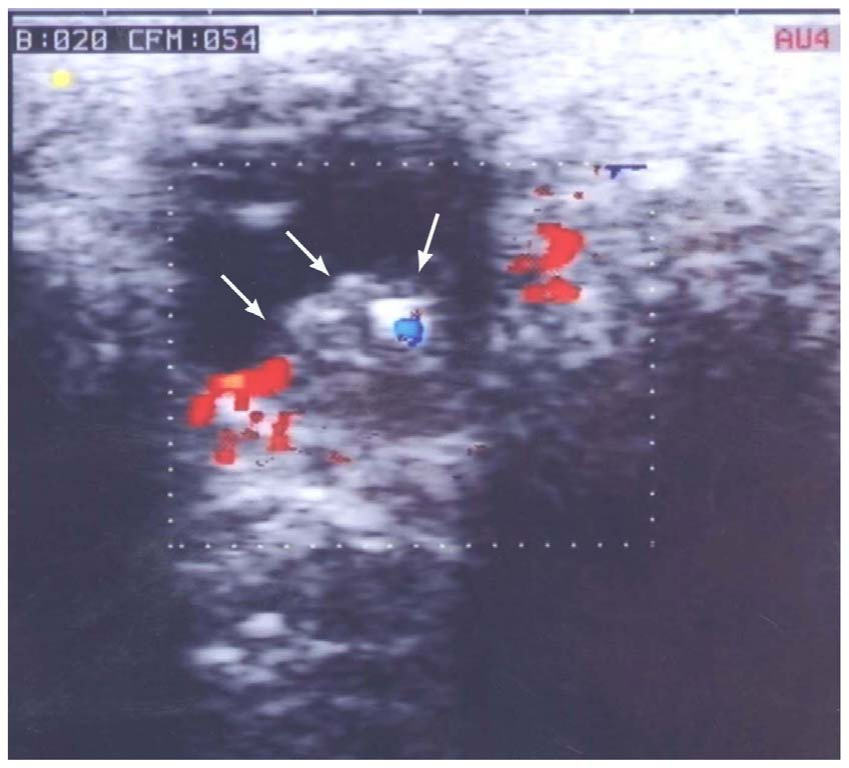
Color Doppler ultrasound examination showing an intraocular tumor, 8× 6 mm, with rich blood flow within the mass.
